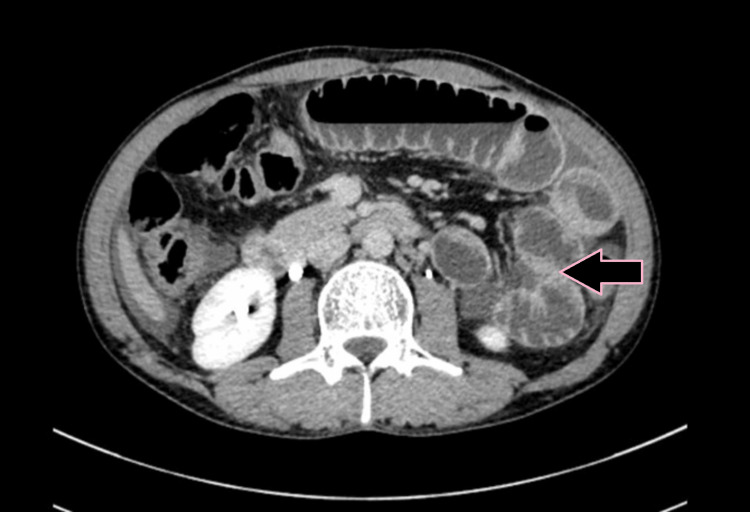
Axial DECT image showing circumferential mural thickening of jejunum with luminal narrowing (case 1). DECT: dual-energy computed tomography
